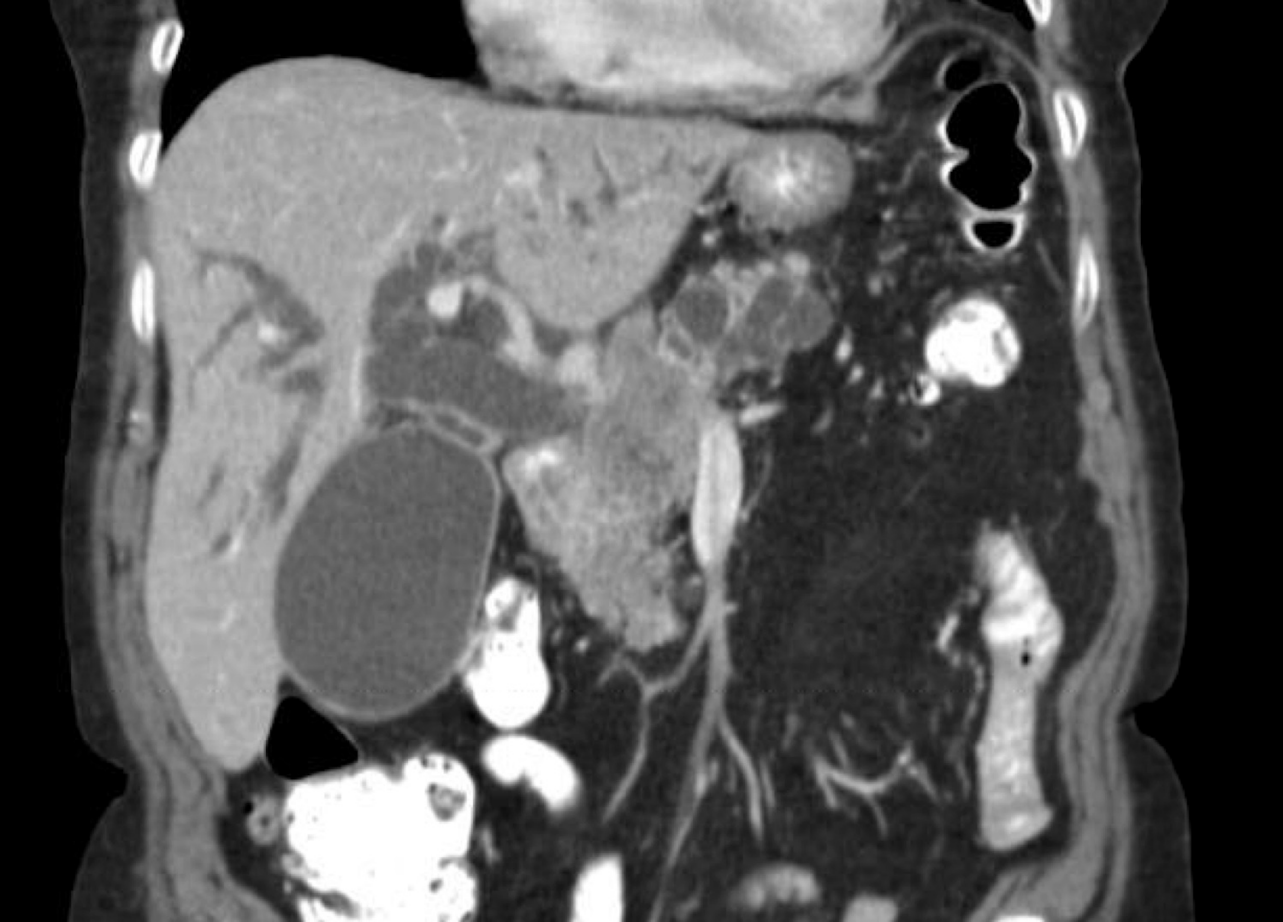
CT Abdomen with Contrast CT scan with contrast demonstrating large pancreatic head mass causing biliary obstruction with extensive intrahepatic and extrahepatic ductal dilatation and a distended gall bladder.
